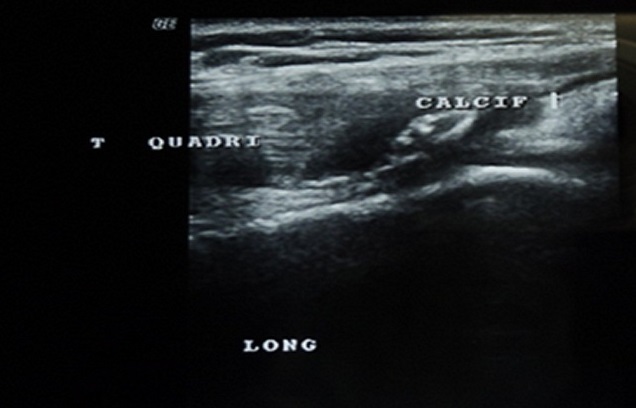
Ultrasound showing rupture of the quadriceps tendon with calcifications
